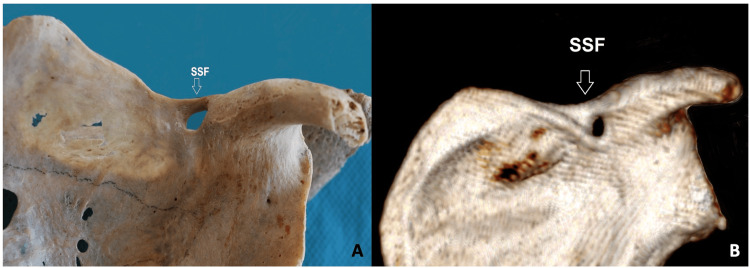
The SSF variant. A. On a dry scapula. B. On a computed tomography scapula. SSF, suprascapular foramen
